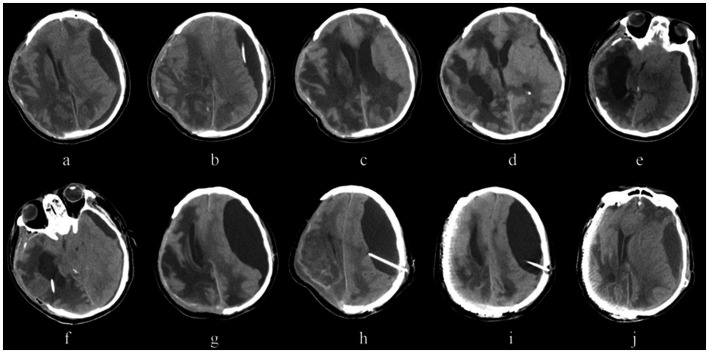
Serial CT images from a representative case. (a) Initial head CT scan on admission shows status post‑right frontotemporoparietal decompressive craniectomy, with a subdural effusion over the left frontotemporoparieto‑occipital region causing a 13 mm midline shift to the right. (b) Head CT after left parietal burr‑hole drainage of the subdural effusion. (c) Follow‑up CT showing gradual reduction of the subdural effusion and evidence of bilateral ventricular dilation. (d) CT performed one week after removal of the subdural drain reveals recurrence of the subdural effusion and significant enlargement of the right temporal horn of the lateral ventricle. (e) Repeat CT three days later shows persistent left frontotemporal subdural effusion and further enlargement of the right temporal horn. (f) CT following placement of a right temporal horn ventriculoperitoneal shunt. (g) Over the subsequent two weeks, CT demonstrates progressive enlargement of the left frontotemporoparietal subdural effusion, with no improvement after ventriculoperitoneal shunt valve pressure adjustments. (h) CT after placement of a subdural‑peritoneal shunt. (i) CT obtained one month later, after the patient’s condition had stabilized and cranioplasty was performed. (j) CT one month post‑cranioplasty shows gradual decrease of the subdural effusion; both hydrocephalus and subdural effusion are well‑controlled.
